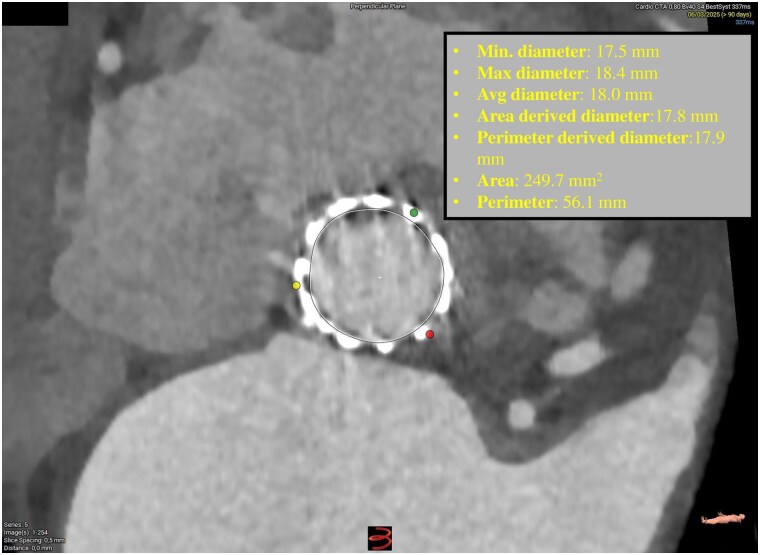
Aortic anulus dimensions at computed tomography. Avg, average.
